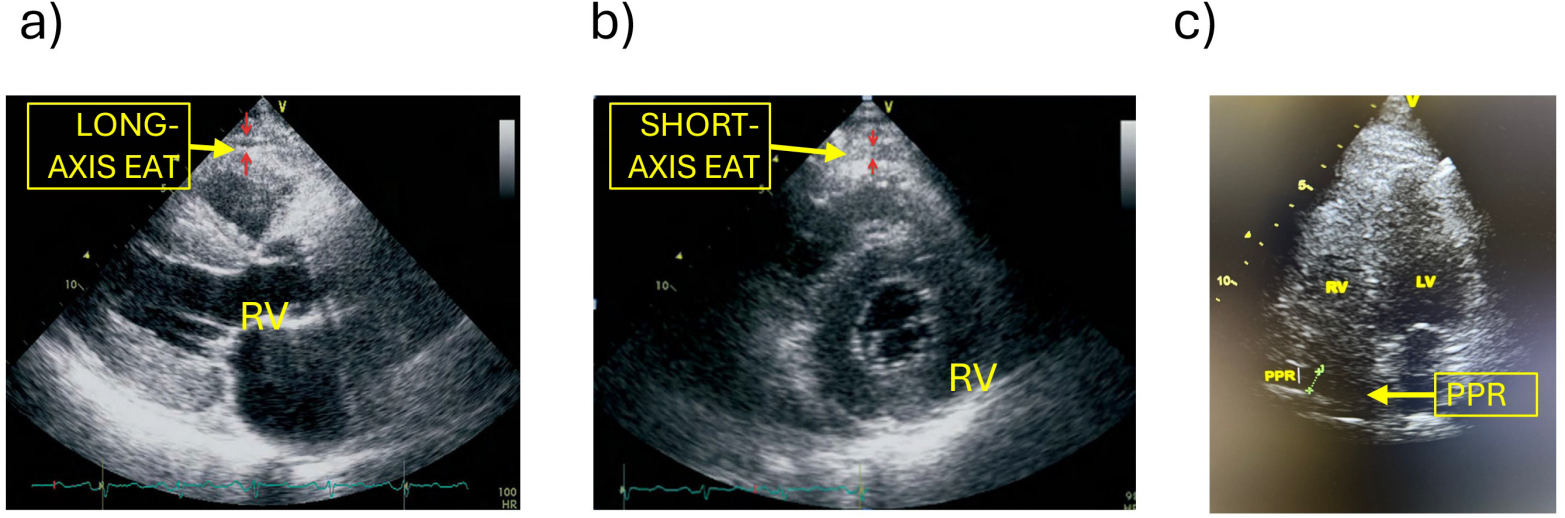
Ultrasound images corresponding to the three EAT measurements (a) long-axis, (b) short-axis, and (c) PPR. EAT, Epicardial adipose tissue; LV, left ventricle; PPR, posterior cardiac (pericardic) recess; RV, right ventricle. .
